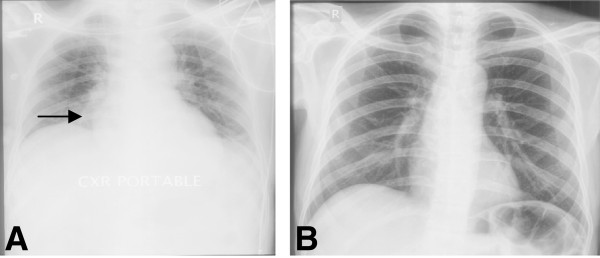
Chest X-ray before and after treatment for Nocardia (Case 3). (A) Pre-treatment consolidation right anterobasal segment & pleural effusion. (B) Post-treatment resolution of right anterobasal segment consolidation and pleural effusion.
